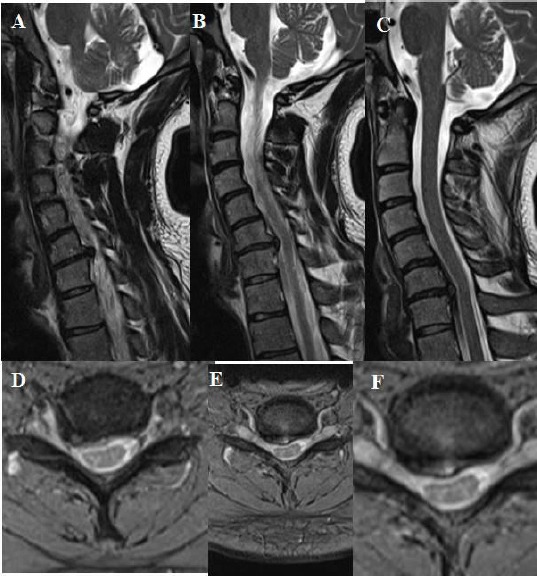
A, B, C, D, E, F: T2 weighted sagittal and axial MRI showing one level cervical root compression due to soft cervical disc extrusion on the right side between cervical 6 and cervical 7. In addition, sagittal MRI showing cervical kyphosis, and cervical lordosis angle was measured 10 degrees on lateral X-ray at the preoperative period*.
